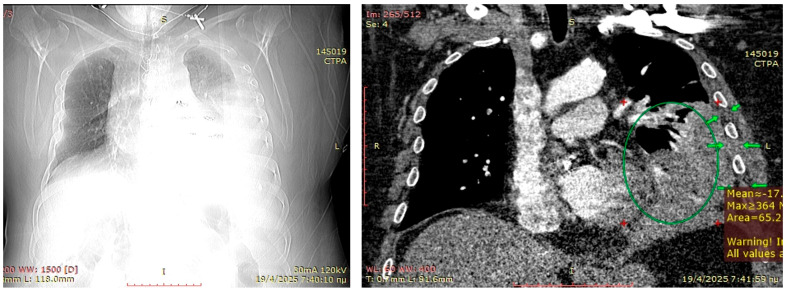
Preoperative planning CT-scan. Although the topogram similar to a standard antero-posterior chest x-ray shows the approximate extent of a parapneumonic effusion and an estimation of the location, it is of little practical value compared to a CT-scan. The right coronal view of the CT-scan is of much higher value to recognize the severe parenchymal infiltration (green circle) which prohibits decortication and describes the thickness of the chest wall to estimate if a Sawamura thoracoplasty by soft-tissue mobilization can be attained (green arrows).
